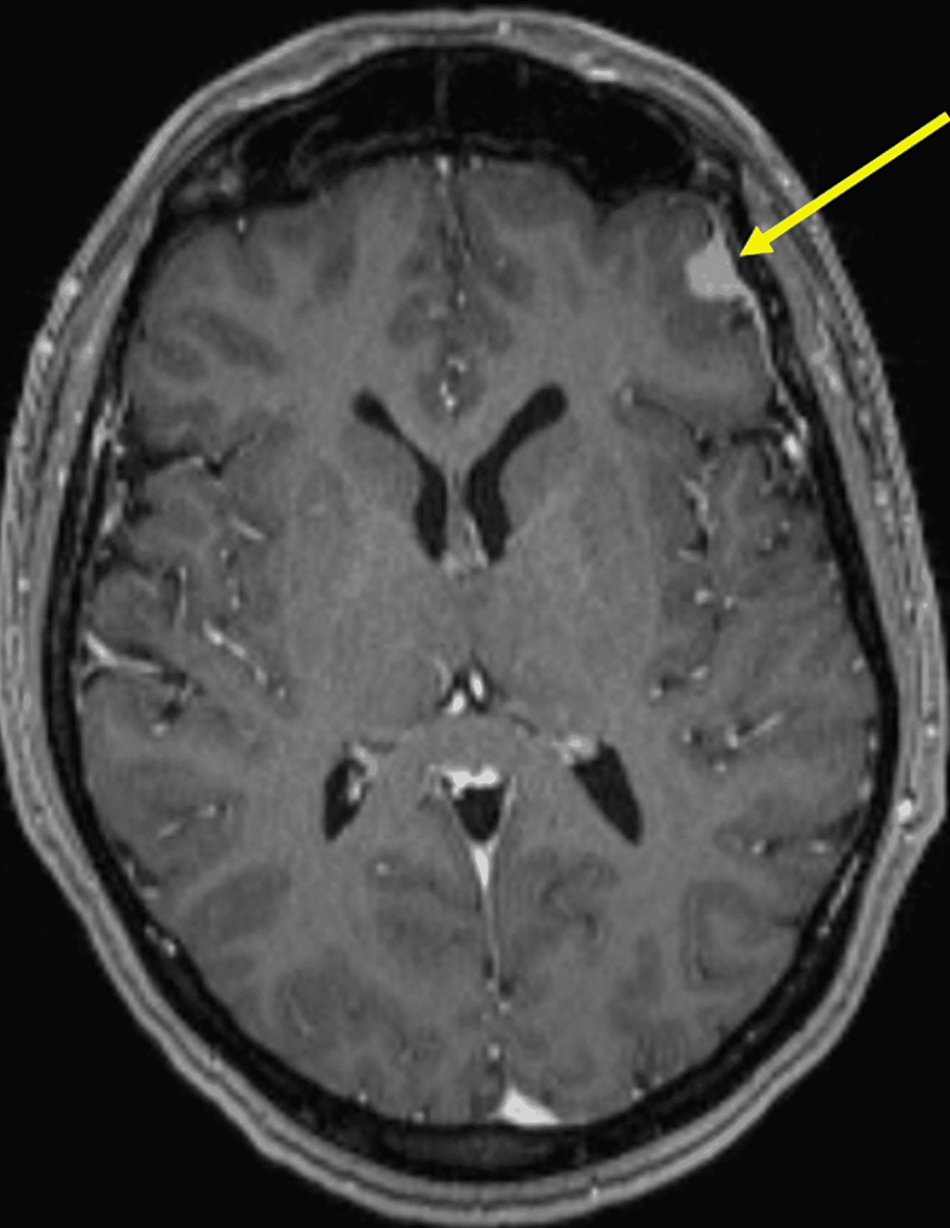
Brain MRI showing a left frontal extra-axial mass (1.0 x 0.8 cm) confirmed to be a meningioma on pathology
